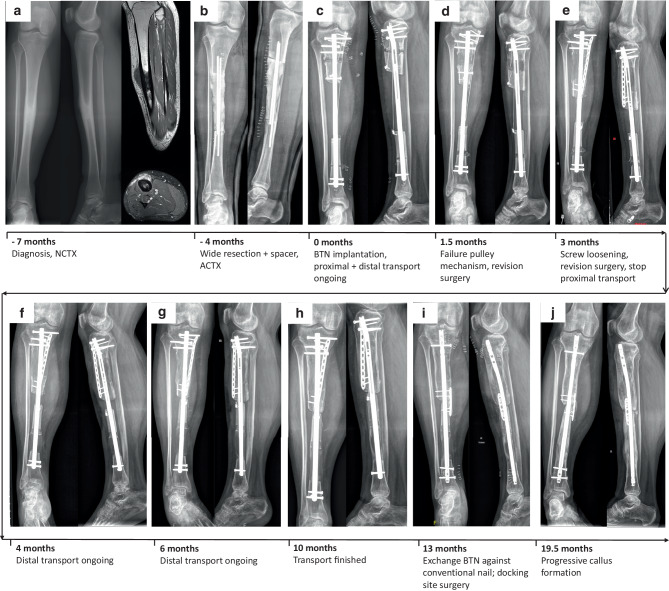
Clinical course of patient 1 (18-year-old male patient with Ewing’s sarcoma of right tibial diaphysis. Images of patient 1 showing (a) preoperative x-rays in anterior-posterior (a.p.) and lateral planes together with two magnetic resonance imaging (MRI) scans in sagittal (top) and axial (bottom) of the right tibia. X-ray images in a.p. and lateral planes following wide resection and spacer implantation (b), directly after implantation of the bone transport nail (BTN; c), at 1.5 months following BTN implantation when the pulley mechanism failed (d), at 3 months when another revision surgery due to screw loosening became necessary (e), at 4 (f) and 6 months (g) with bone transport ongoing, at 10 months with finished bone transport (h), at 13 months when the BTN was exchanged against a conventional nail (i), and at last follow-up (19.5 months) when progressive callus formation was visible, but no complete bony consolidation had taken place (j). (*ACTX* adjuvant chemotherapy,* BTN* bone transport nail;* NCTX* neoadjuvant chemotherapy)
